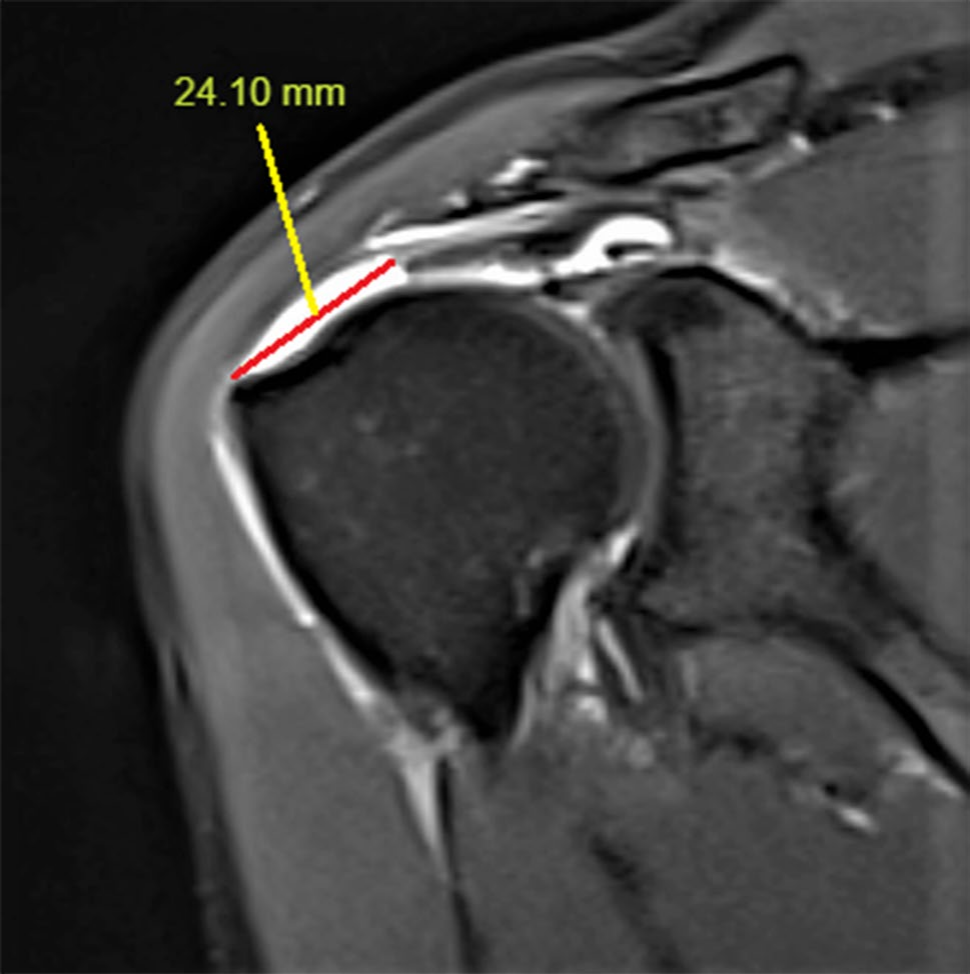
Supraspinatus tendon retraction measurement on a T2-weighted oblique coronal MRI slice. 24.1 mm retraction was measured in the given example
